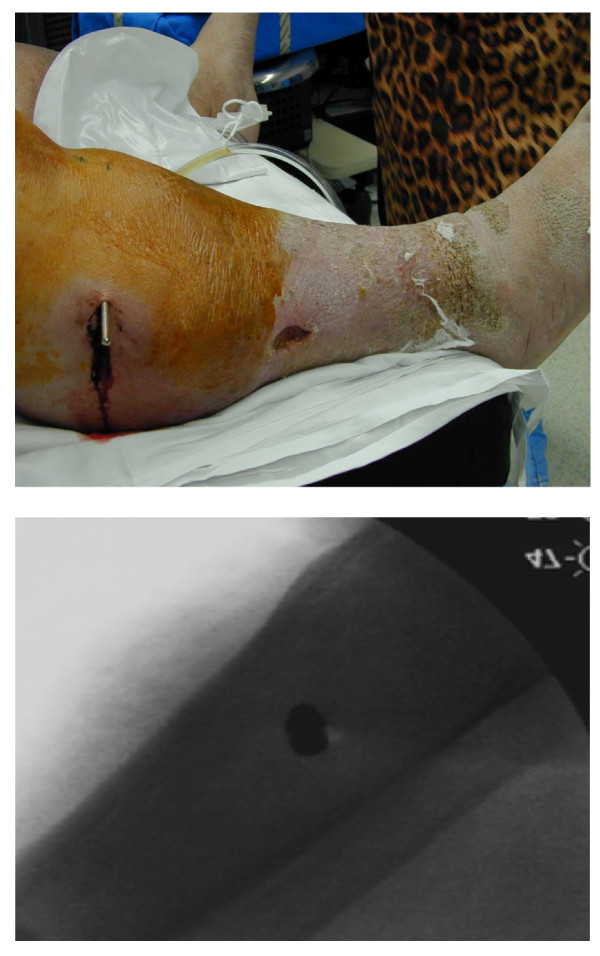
A-B Picture and radiograph of lower extremity with skeletal tibial traction pin in place.
